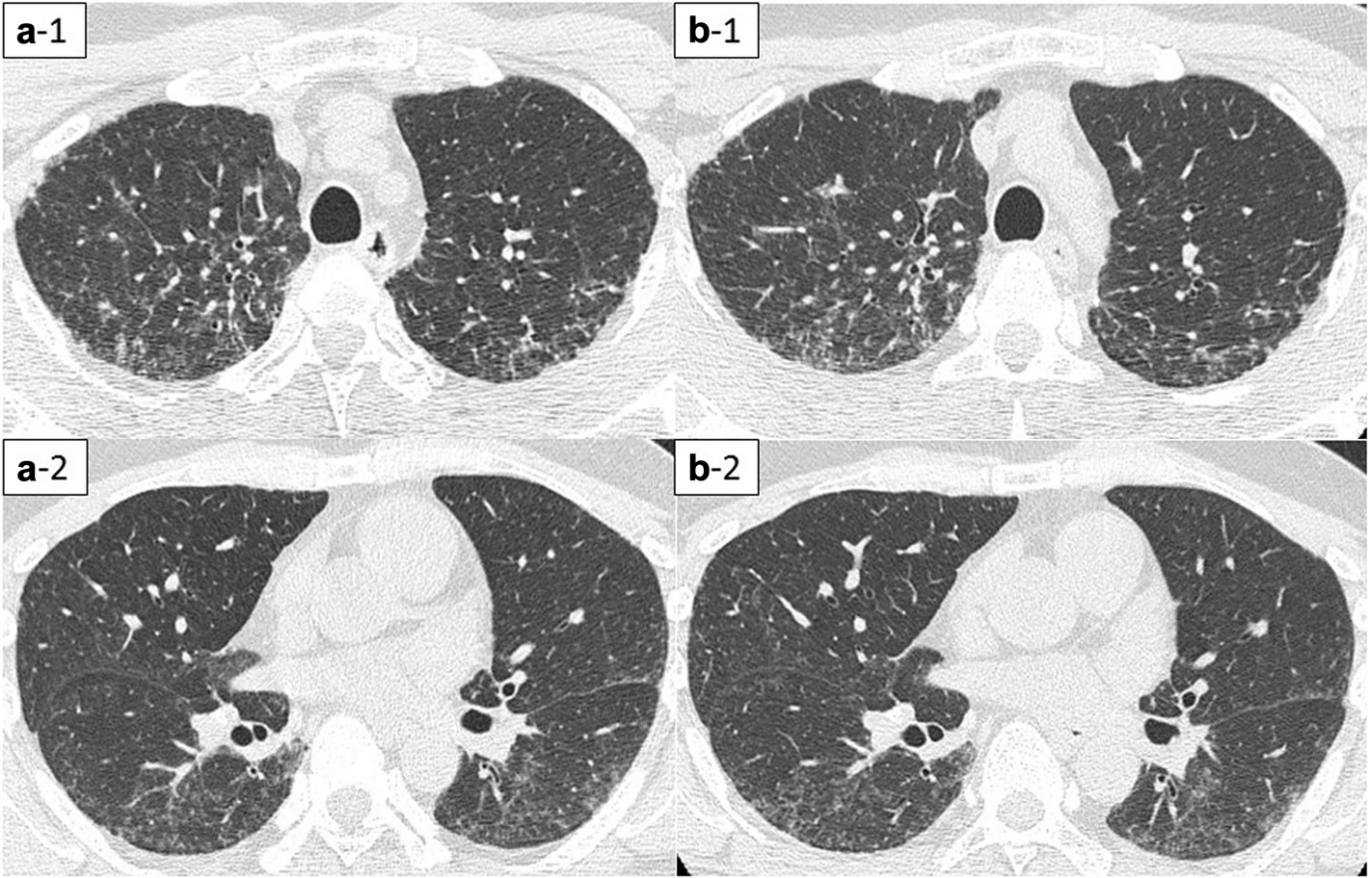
HRCT findings before and after the avoidance of exposure in Case 2. Bilateral diffuse centrilobular micronodular opacities and reticular shadows. a: before the avoidance (a-1: upper lobe; a-2: lower lobe); b: after the avoidance (b-1: upper lobe; b-2: lower lobe)
